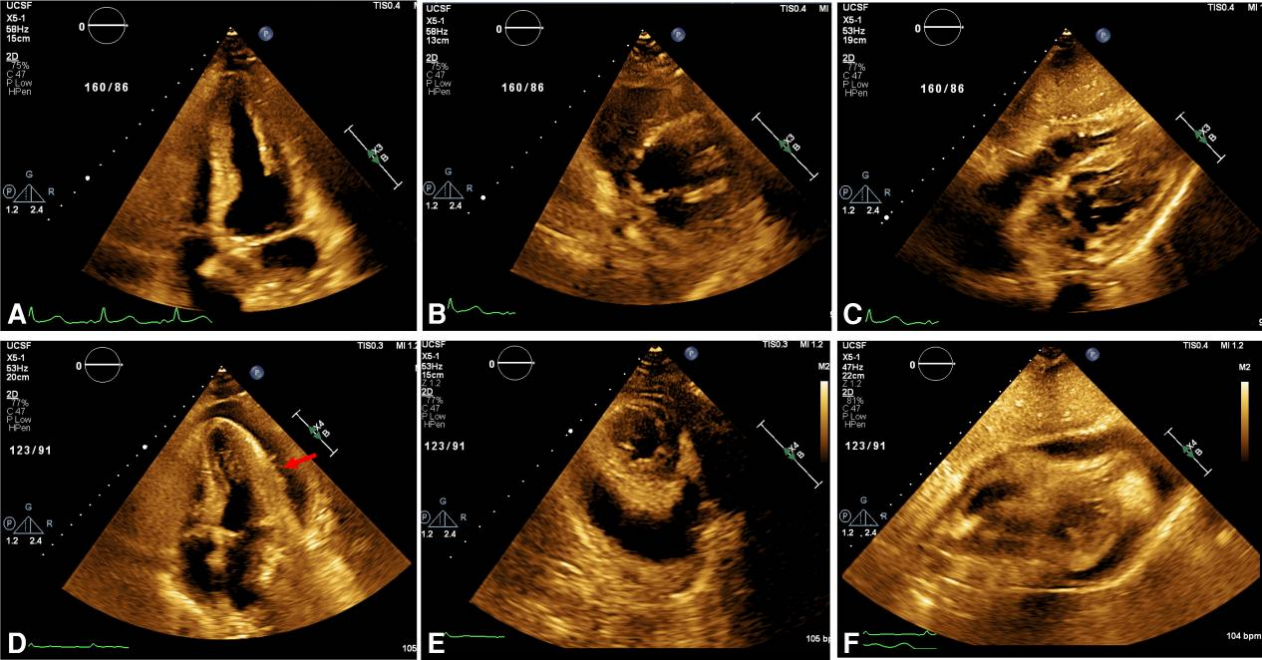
Transthoracic echocardiogram with new moderate pericardial effusion. Baseline pre-radiation therapy (RT) transthoracic echocardiogram without evidence of pericardial effusion: (*A*) apical four-chamber view, (*B*) short-axis view, and (*C*) subcostal view. Transthoracic echocardiogram 6 days after RT showing a new circumferential moderate pericardial effusion with fibrinous strands (arrow). There was no echocardiographic evidence of tamponade: (*D*) apical four-chamber view, (*E*) short-axis view, and (*F*) subcostal view.
